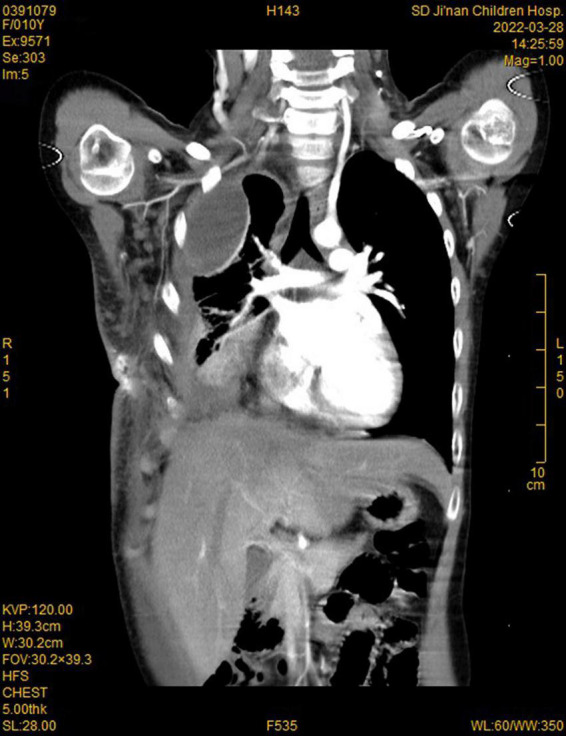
Computed tomography (CT) imaging showed enhancement of chest wall lesions, atelectasis of the right lung, right encapsulated pleural effusion, and pleural thickening.
